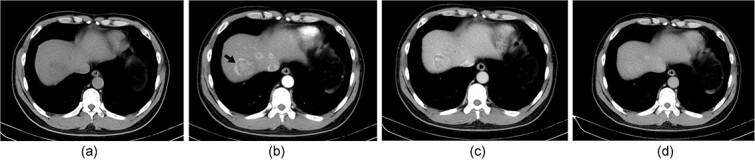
(a) The lesions exhibit low density with unclear margins on non-contrast computed tomography (CT) imaging. (b-d) on dynamic contrast-enhanced CT imaging, centripetal enhancement can be observed. The sizes of lesions varied, with the largest being 4.1 × 4.3 cm in diameter (black arrow), with irregular shape.
